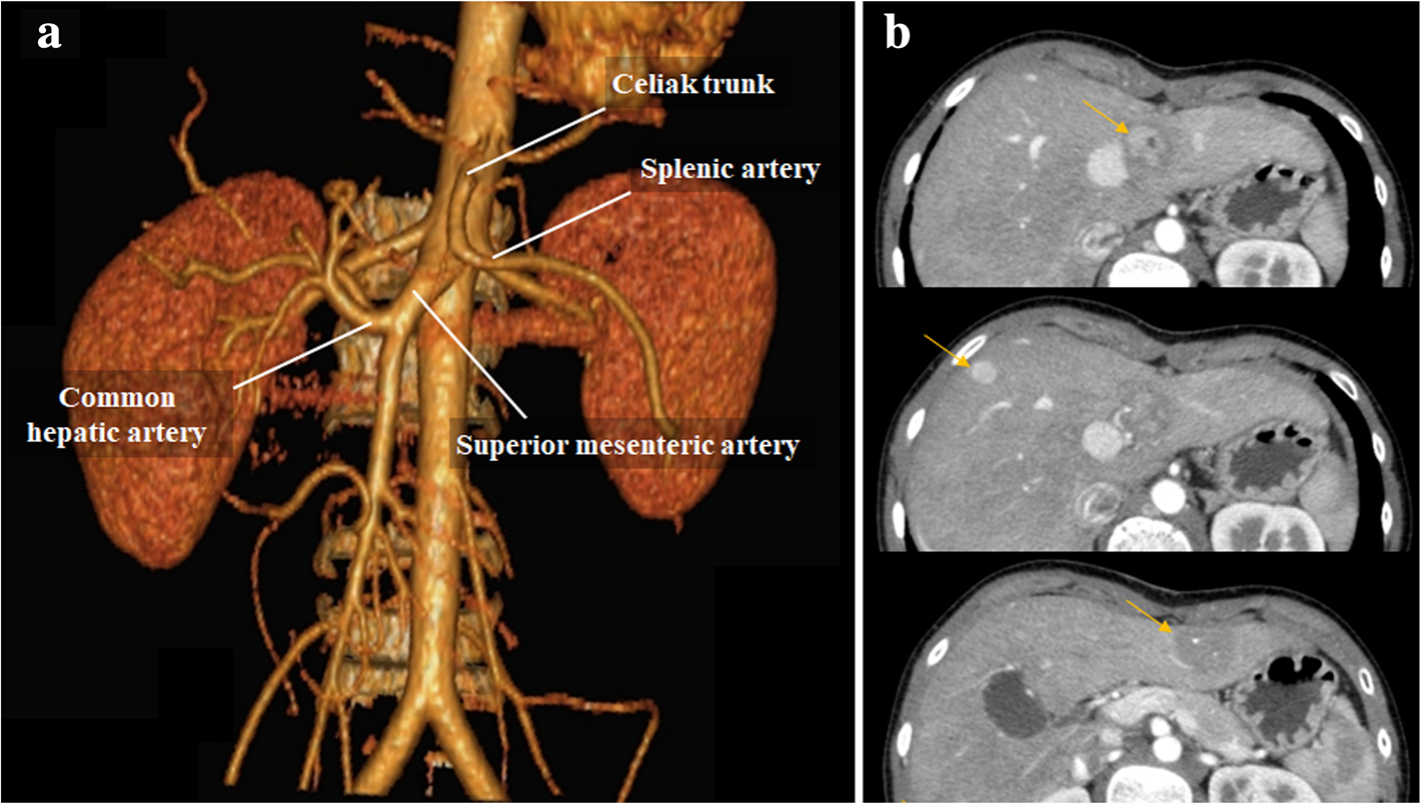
Congenital extrahepatic portosystemic shunt—Abernethy malformation. a VRT image from arterial phase CT of the same patient in Fig. 7. Note aberrant origin of the hepatic artery emerging from superior mesenteric artery. There is also increase caliber of the common hepatic artery and its branches, consequence of the augmented arterial compensatory flow. b Late arterial phase CT images reveal multiple liver nodules (orange arrows), frequently associated with Abernethy malformation [12]
